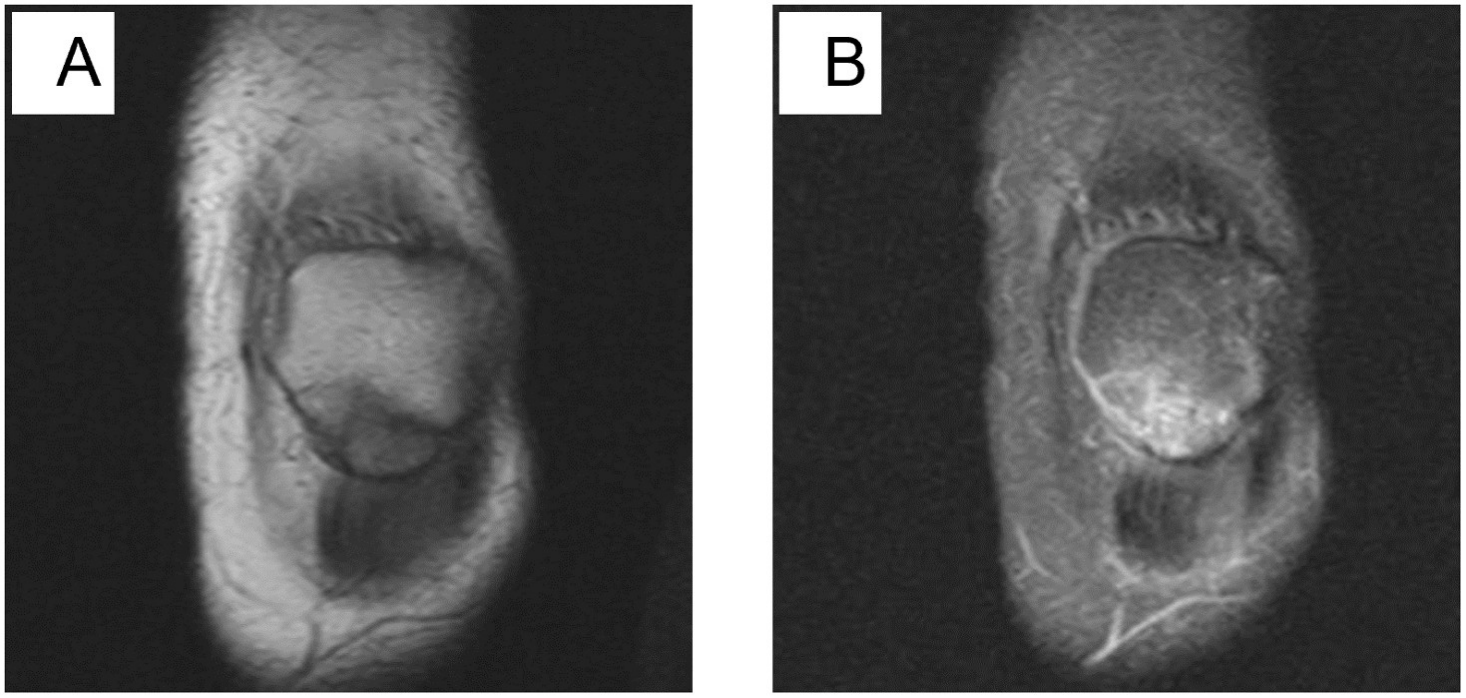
A: Coronal T1-weighted MR image; B: Coronal fat-suppressed proton density-weighted MR image. Female, 32 years old, transverse fracture. The patellar was divided into two parts: upper and lower.
